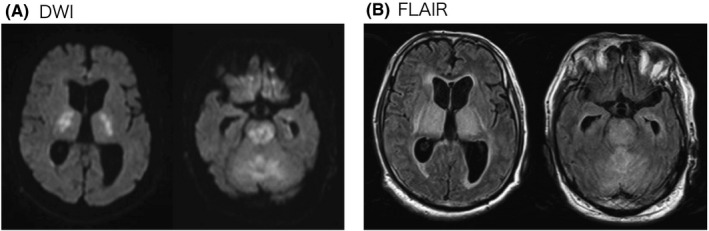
Head magnetic resonance imaging. (A) Diffusion‐weighted imaging (DWI) and (B) fluid‐attenuated inversion recovery (FLAIR) followed by the second head computed tomography. Both images show bilateral symmetric lesion of the thalamus, cerebellum, and brain stem.
